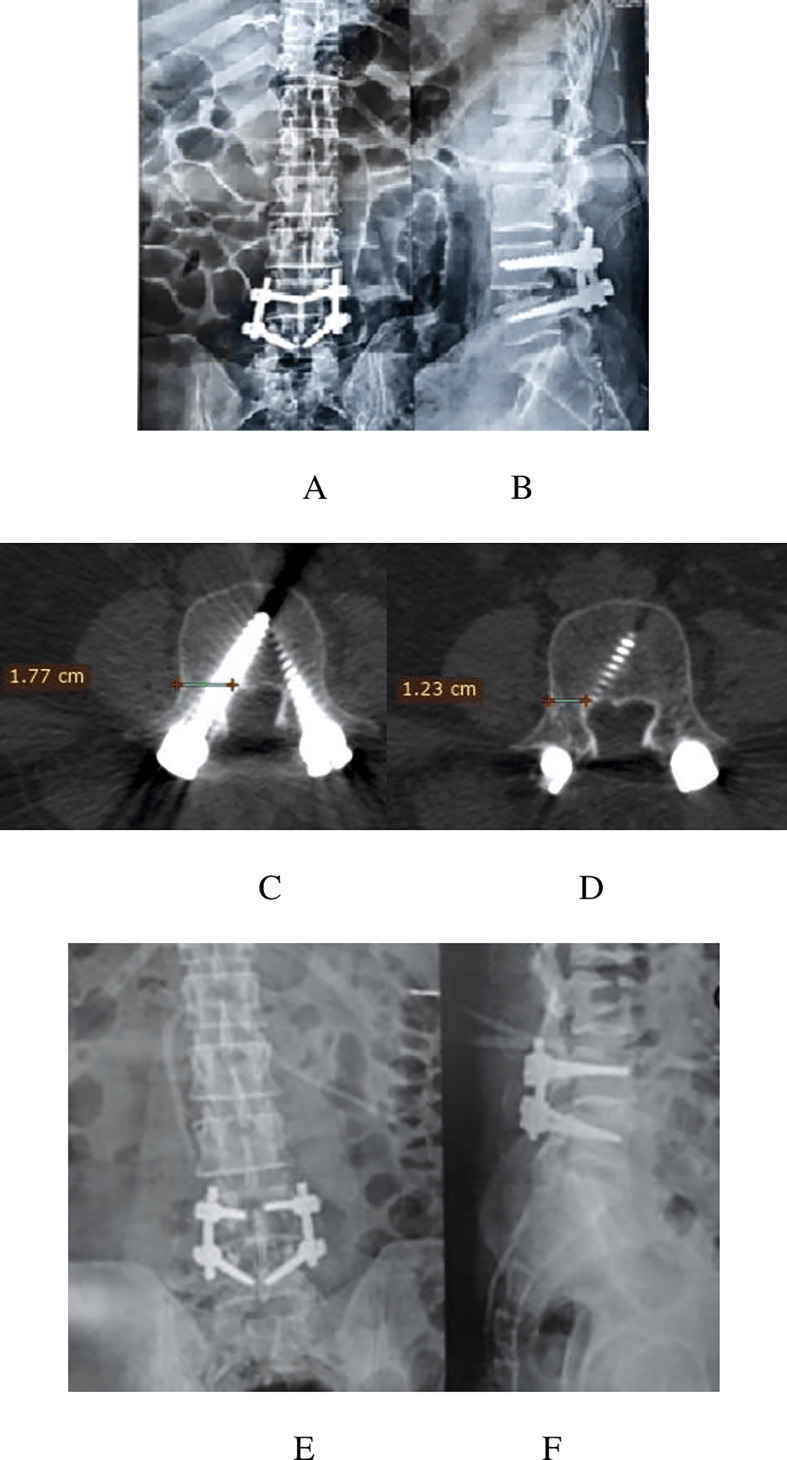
Plain X-ray images of the LSS AP (A), lateral (B), and axial (C) MSCT images showing a 5.4 mm medial violation of the L4 screw (measured by subtraction of 1.23 cm in Figure D from 1.77 cm in Figure C), plain X-ray images of the LSS AP (E) and lateral (F) after revision, and examination of the intraoperative medial wall of the pedicle and ensuring that no wall placed the screw. Therefore, there is no need for postoperative MSCT.
